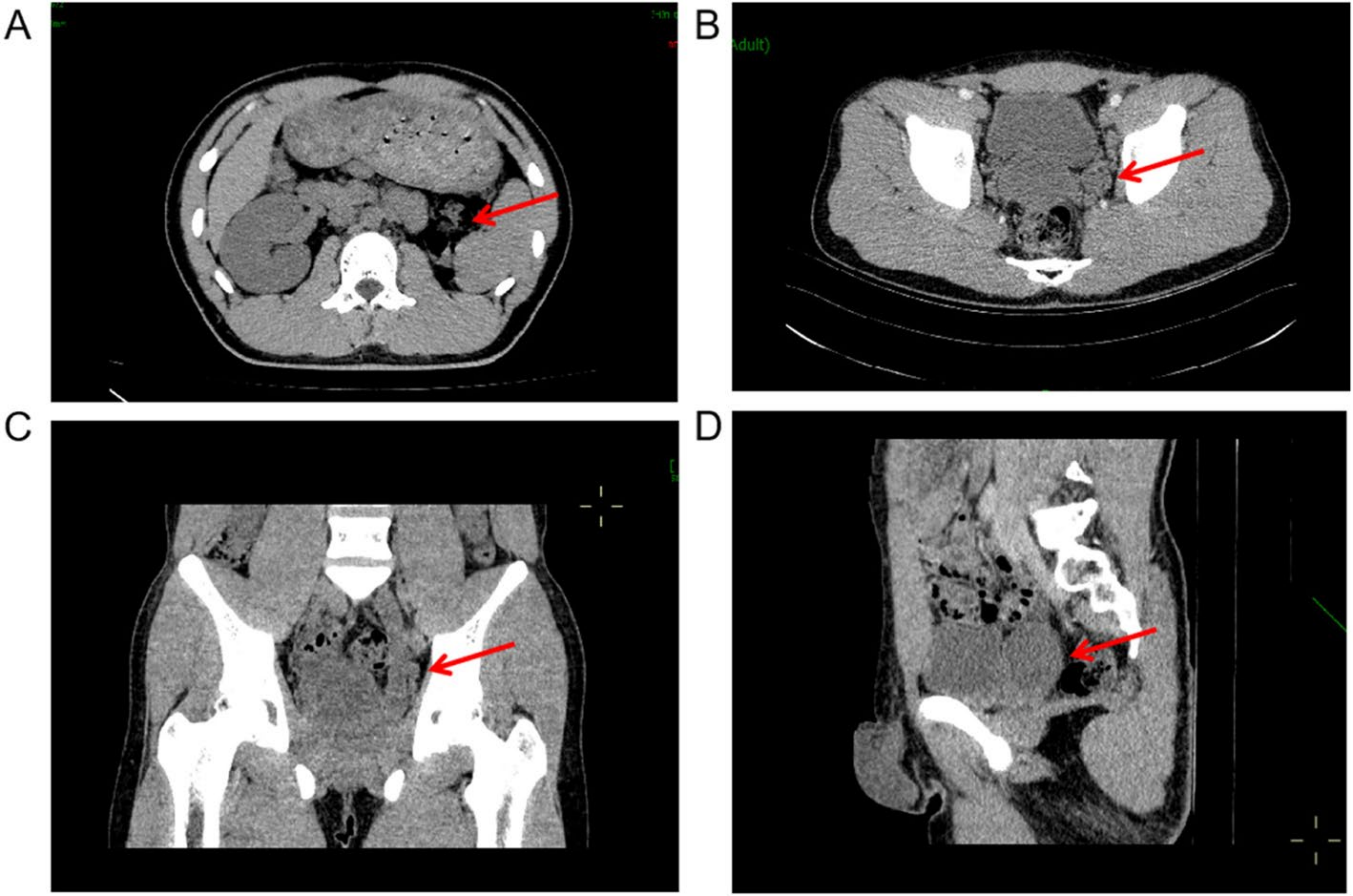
Computed tomography findings in the patient. A An axial abdominal CT plain scan highlighted the absence of the left kidney with a red arrow. B An axial pelvic CT-enhanced scan revealed a cystic low-density shadow approximately 50 mm in size located posterior to the bladder, along with a notably widened lower segment of the left ureter, as indicated by the red arrow. C A coronal CT plain scan showed both the cystic low-density shadow and the expanded ureter on the left side, as noted by the red arrow. D A sagittal CT plain scan delineated the cystic low-density shadow situated posterior to the bladder, marked by the red arrow
